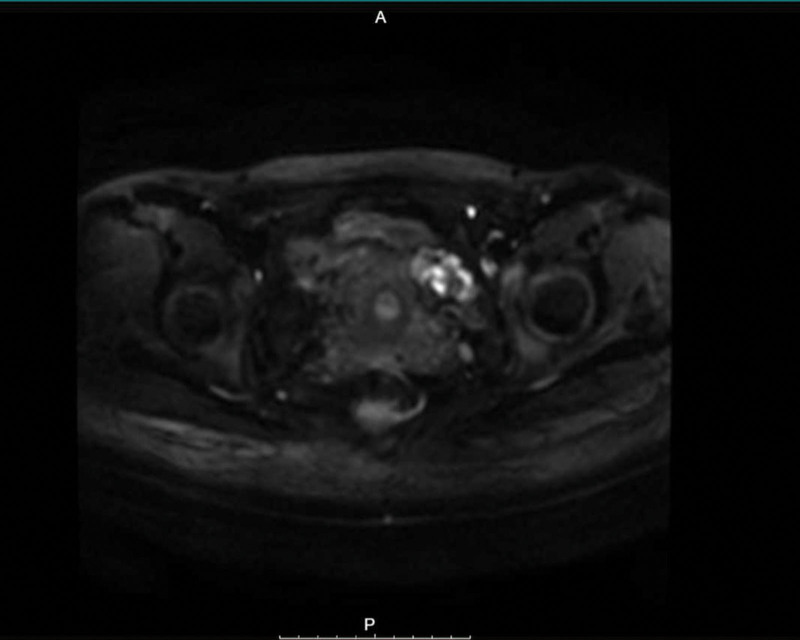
First postoperative MRI image.
